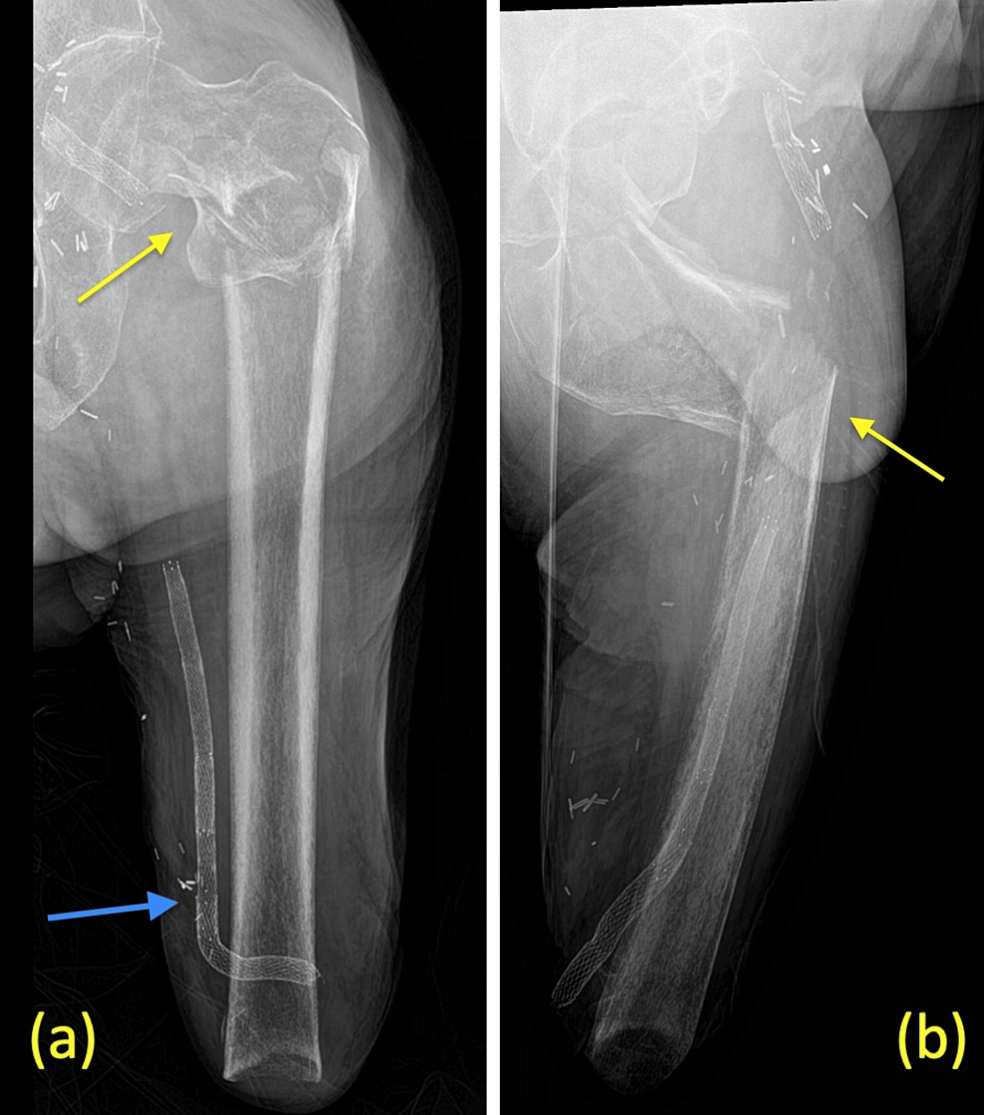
Radiograph imaging a: anteroposterior view of the left femur showing a comminuted intertrochanteric fracture with subtrochanteric extension (yellow arrow), amputated femur, and vascular graft (blue arrow); b: lateral view of the left femur showing anterior displacement of the fracture.
